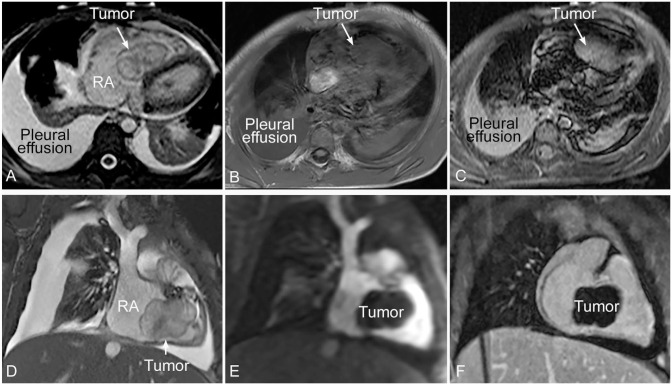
MRI images showing encapsulated mobile tumor in the cavity of the right atrium and ventricle across the tricuspid valve. It is attached to the inlet part of the ventricular septum. The tumor shows heterogeneous signal intensity on steady state free precession (SSFP) images (A and D). It is a low signal intensity lesion at T1-wieghted imaging (B) and high signal intensity lesion at T2-weighted imaging (C). The lesion does not show significant enhancement at perfusion imaging (E) and late gadolinium enhancement imaging (F).
